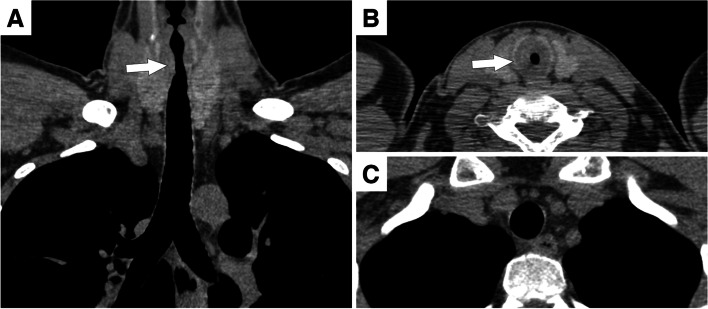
Granulomatosis with polyangiitis. A Coronal reformation of the unenhanced chest computed tomography shows focal tracheal wall thickening (arrow). B On the axial image, the tracheal wall thickening (arrow) is circumferential and responsible for lumen stenosis. C Below, the thoracic trachea is normal
